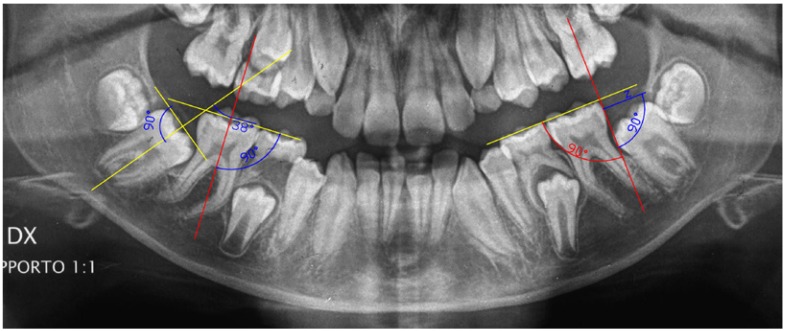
Panoramic radiograph , with a magnification rate of 1:1 , at the time of one third of MM2 root formation (T1): The measurements of the angle of inclination of MM2 ( right side ) and of the distance from the distal height of the contour of the first mandibular molar (MM1) to the anterior margin of mandibular ramus (left side) are shown.
